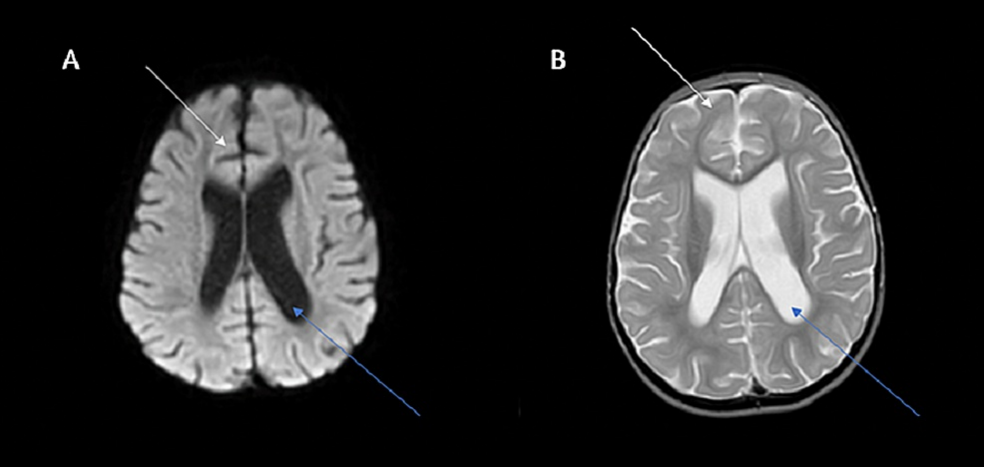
Image A: T1 MRI sequence, axial view Image B: T2 MRI sequence, axial view: both images show ex vacuo ventriculomegaly (blue arrows) and frontal atrophy (white arrows). MRI: magnetic resonance imaging
